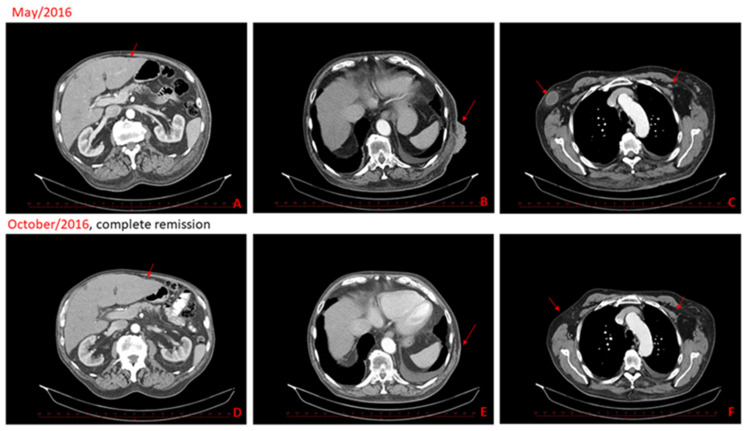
CT scans from May 2016 show progressive disease, new liver metastasis (A), skin and subcutaneous metastasis (B), left subclavicular node metastasis and right node axillary metastasis (C). CT scans in the second-line show complete remission of the metastatic disease, two months after cryotherapy and half a year after the last dose of ipilimumab (D–F).
